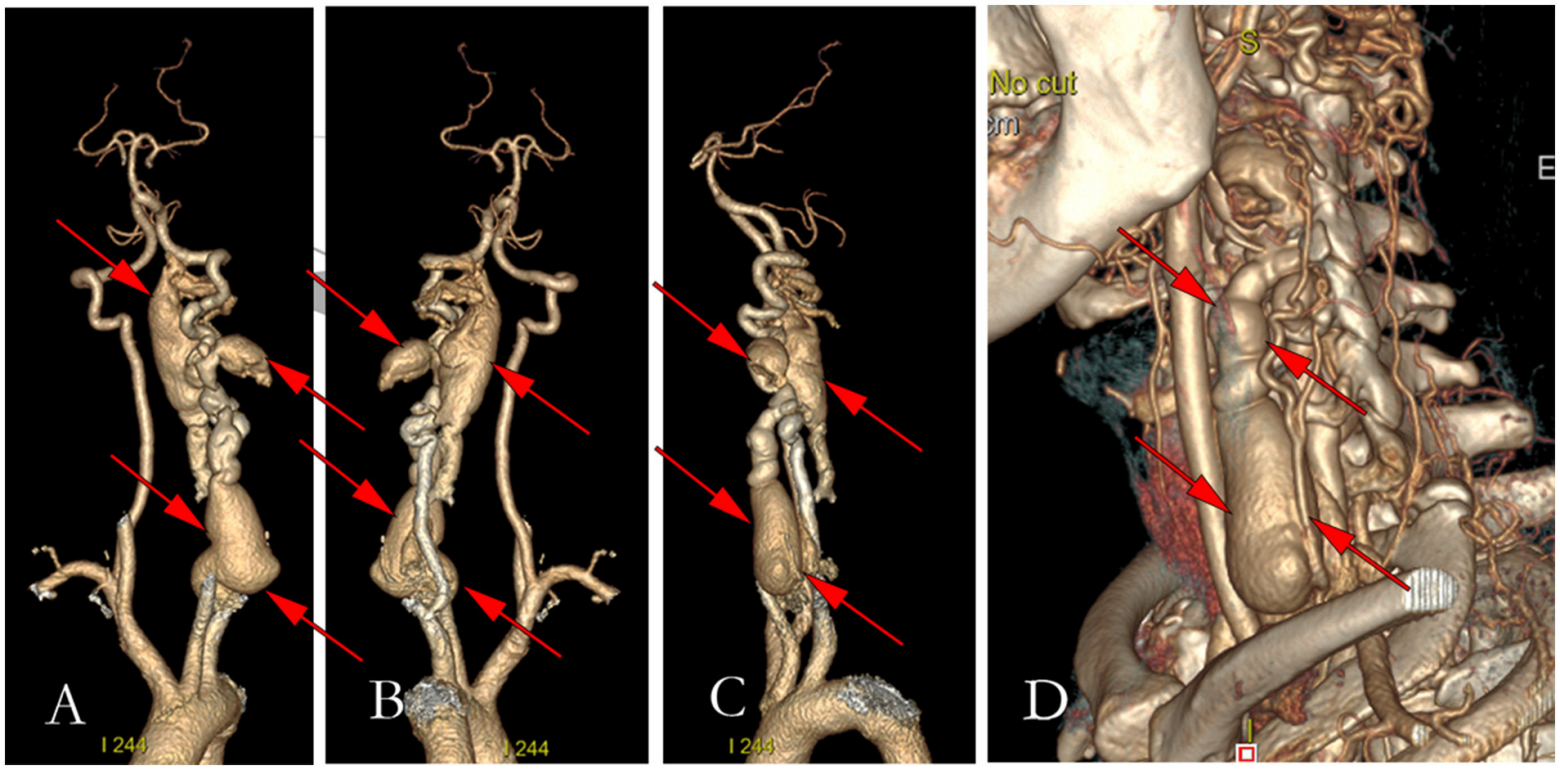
Figures of preoperative three-dimensional computerized tomography. (A) The anterior view of the three-dimensional CT of vertebral arteriography; (B) The left view of the three-dimensional CT of vertebral arteriography; (C) The post view of the three-dimensional CT of vertebral arteriography; (D) Three-dimensional reconstruction of the left neck.
